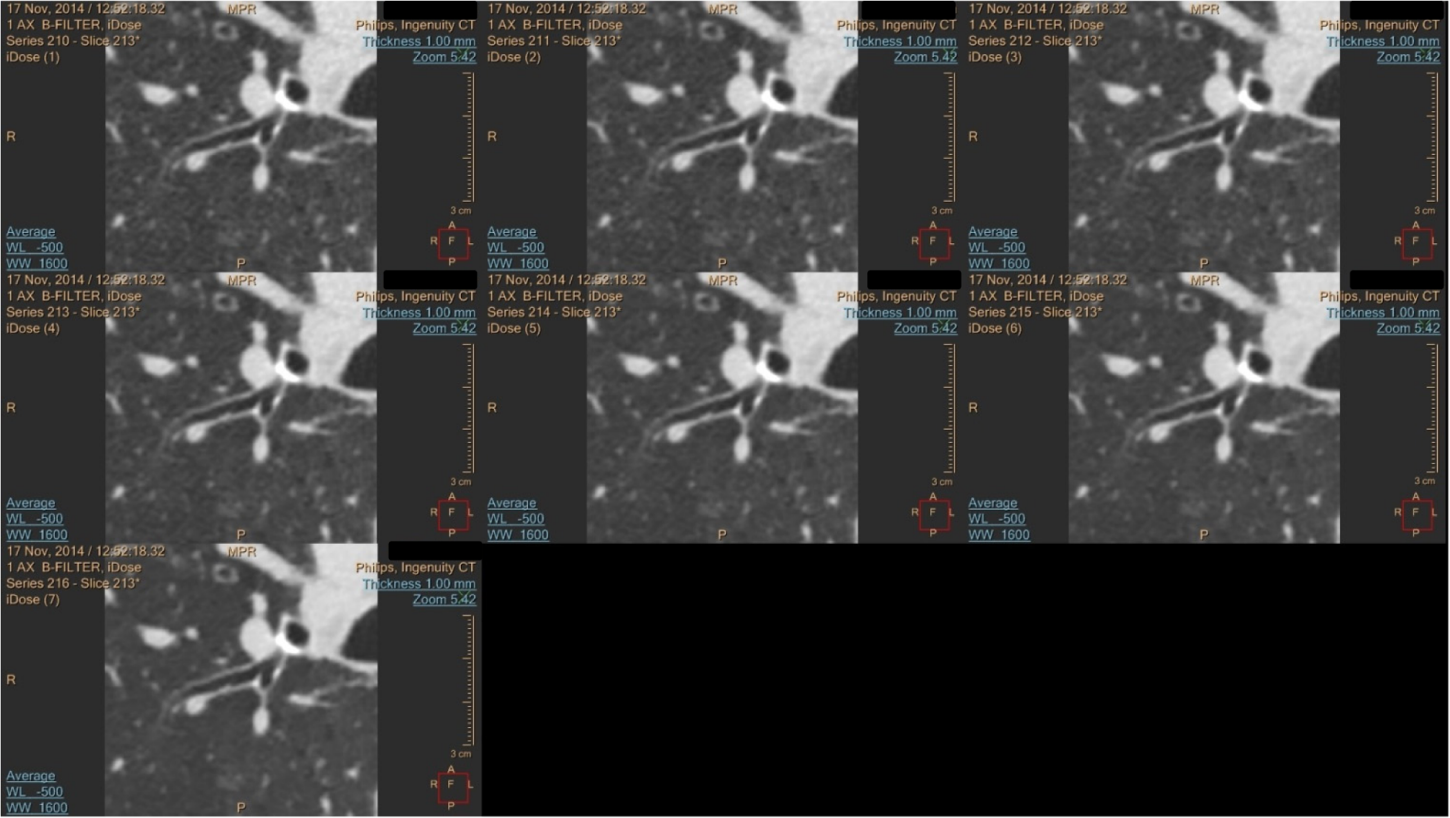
Magnification of the lung parenchyma in a CT scan with the seven different iDose^4^ levels in ISP6: upper row iDose^4^ level 1 to 3; middle row: iDose^4^ level 4 to 6; bottom: iDose^4^ level 7
